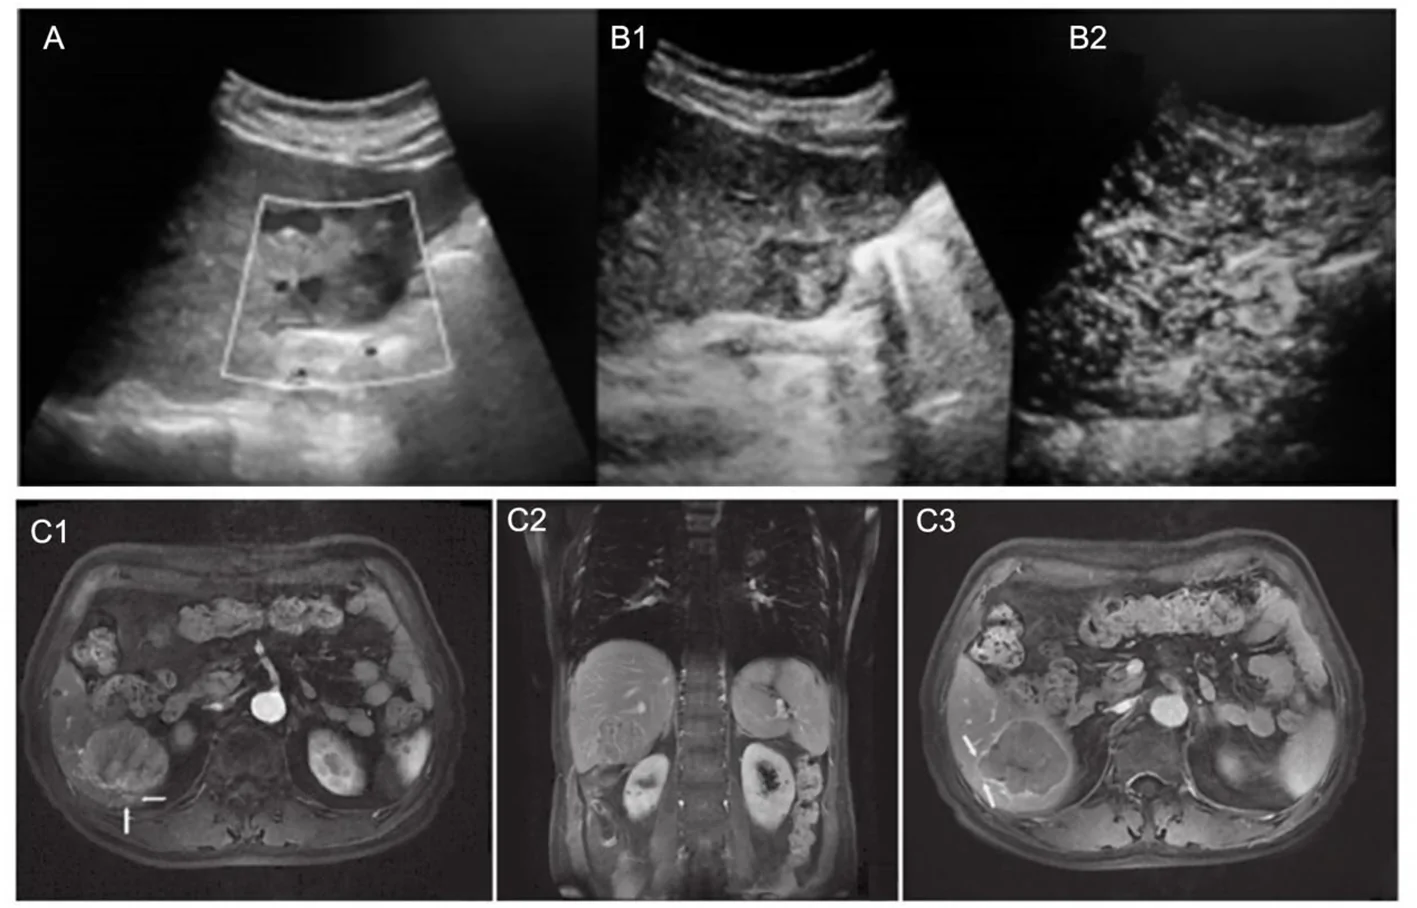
Representative conventional ultrasound, CEUS, and contrast-enhanced MRI findings in a 46-year-old man with HCC recurrence after RFA-TACE. (A) Conventional ultrasound shows blood-flow signals at the margin of the treated area. (B1) Arterial-phase CEUS at 18 seconds shows irregular peripheral enhancement and peritumoral radial enhancement. (B2) Portal venous-phase CEUS at 45 seconds shows contrast washout and hypoenhancement of the lesion. (C1) Arterial-phase MRI at 28 seconds shows peritumoral enhancement surrounding a lesion measuring approximately 4.3 cm. (C2) Portal venous-phase MRI at 60 seconds shows lower lesion enhancement than the adjacent liver parenchyma. (C3) Delayed-phase MRI at 180 seconds shows further contrast washout, an irregular lesion margin, and capsule-like enhancement. CEUS, contrast-enhanced ultrasound; HCC, hepatocellular carcinoma; MRI, magnetic resonance imaging; RFA-TACE, radiofrequency ablation combined with transarterial chemoembolization.
